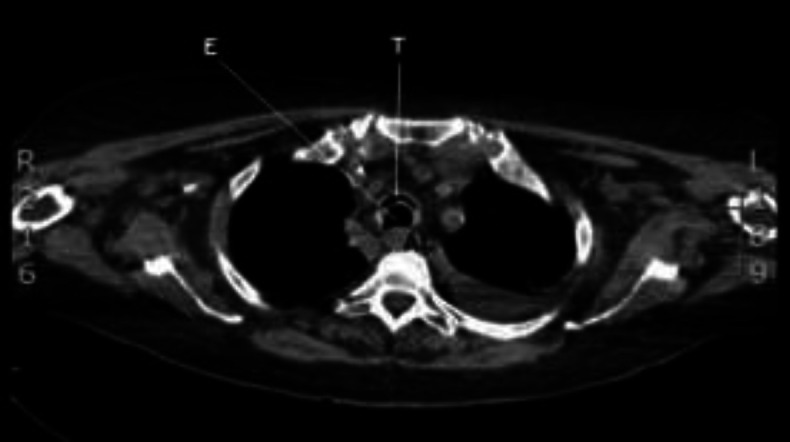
HRCT, axial section (T = trachea, E = esophagus, AL = arteria lusoria)
